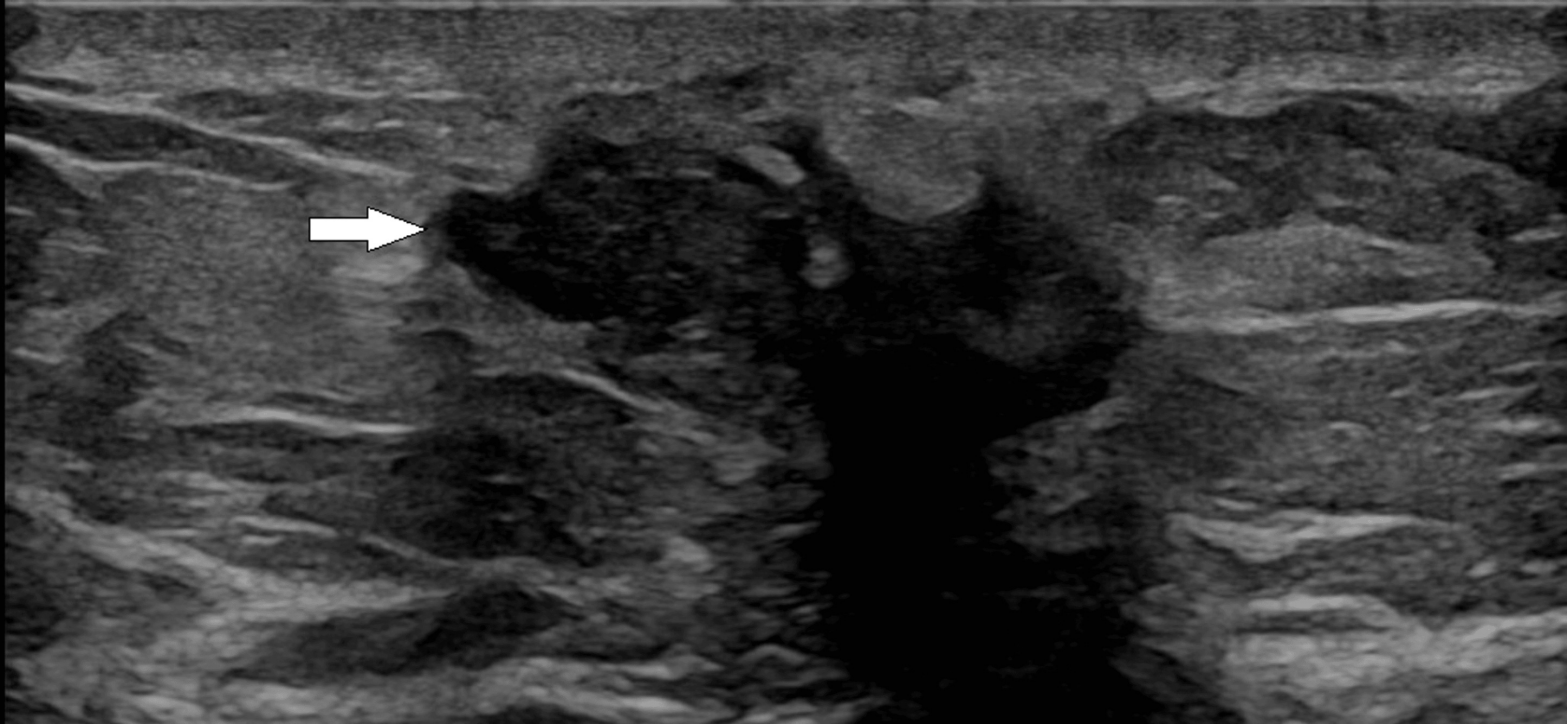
Targeted ultrasound demonstrating an irregular hypoechoic mass with posterior acoustic shadowing corresponding to the mammographic abnormality (arrow).
